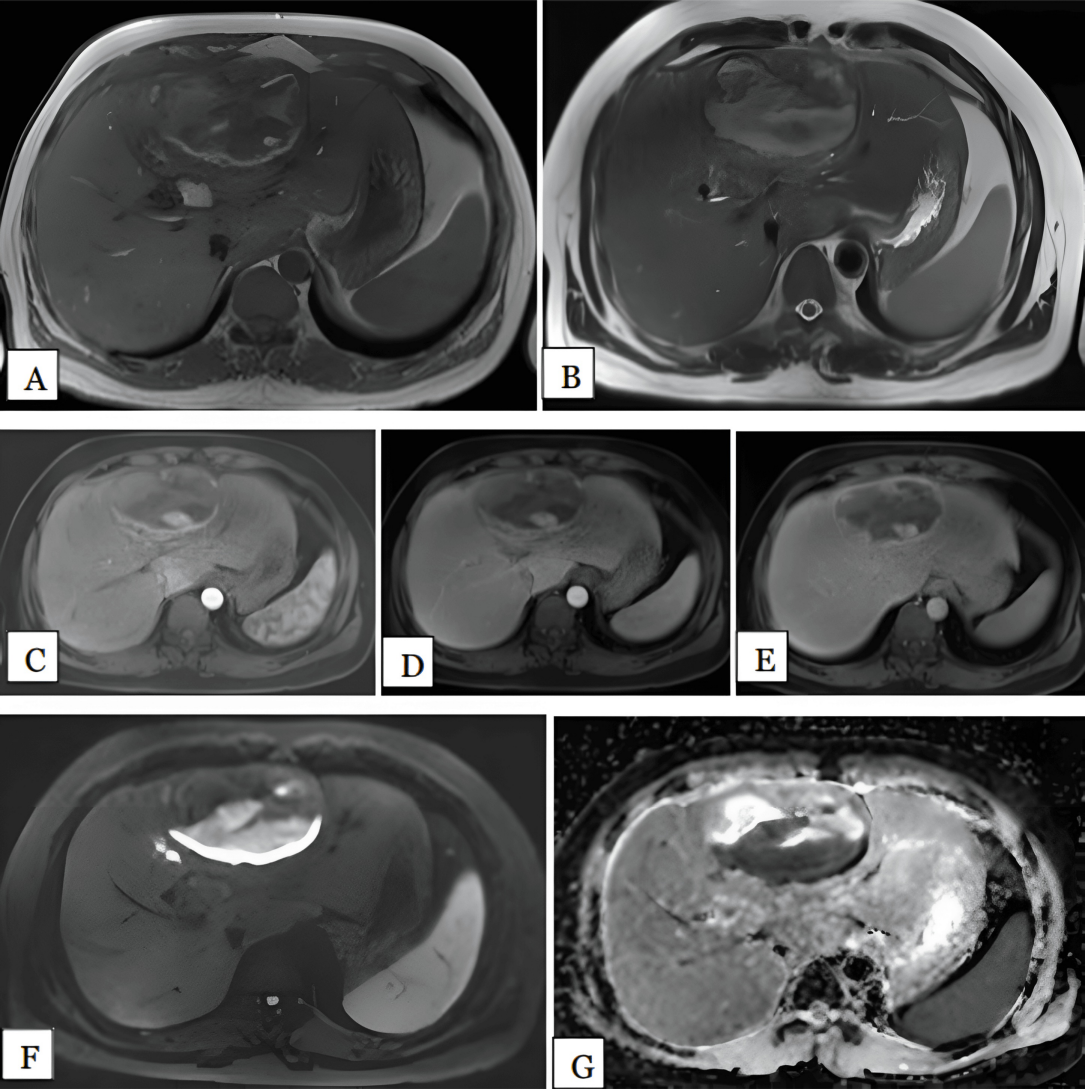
Hematoma in a 36-year-old male Axial T1 (A), T2 (B), arterial (C), portal (D), and delayed (E) phase MRI images show well-defined lobulated lesion. Heterogenously hyperintense on both T1 and T2 measuring 10 x 8 cm were noted involving the segment IVa and IIb. Post contrast showed no enhancement. DWI (F) and ADC (G) maps showed the part of the lesion is hyperintense on DWI with reversal on ADC maps with mean ADC value of 1.1 x 10^-3 ^mm^2^/s, suggestive of partial restriction. DWI: Diffusion-weighted imaging; ADC: Apparent diffusion coefficient
